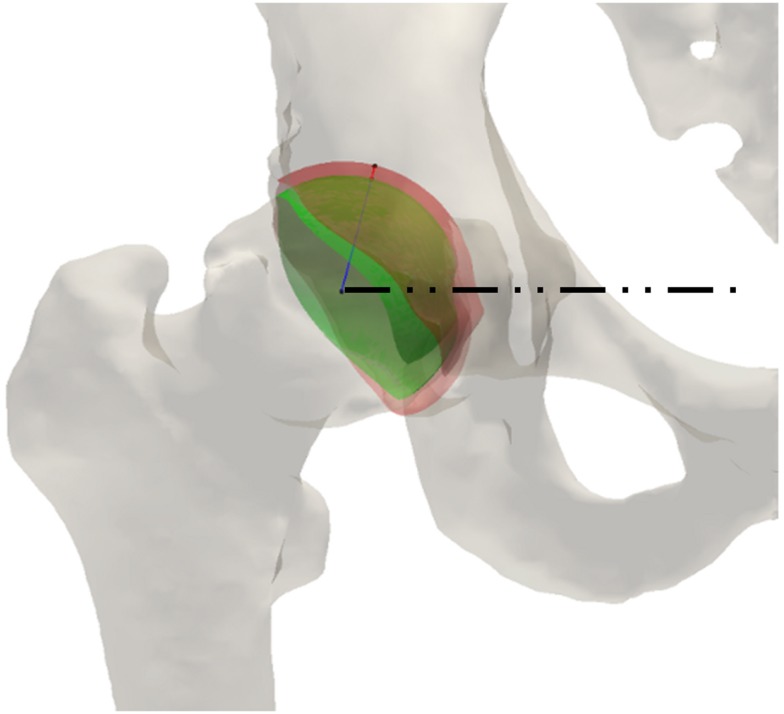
Ray-firing method for indirect computation of cartilage thickness from CT data using bony surfaces. The green and red surface models represent the contact surface on femoral and acetabular sides, respectively. The blue line shows the radial direction from the center of the femoral head to an arbitrary point on the femoral head surface. The red line delineates the radial distance between the femoral head and acetabulum contact surfaces. The dashed line is the medio-lateral axis of the hip, extending through the centers of the femoral heads.
